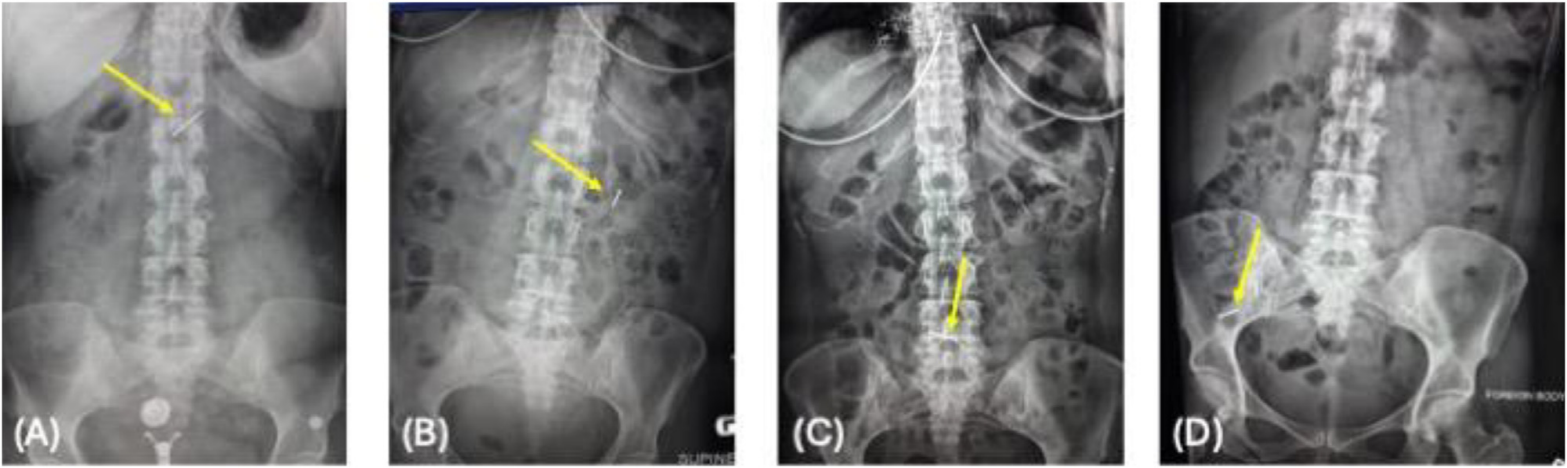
(A) Abdominal X-ray showing a linear radiopaque object in the epigastric region. (B) Follow-up X-ray showing the object in the left upper quadrant. (C) Further progression of the object into the lower abdomen. (D) Radiograph showing the object in the right lower quadrant. Yellow arrows indicate the object in all images.
